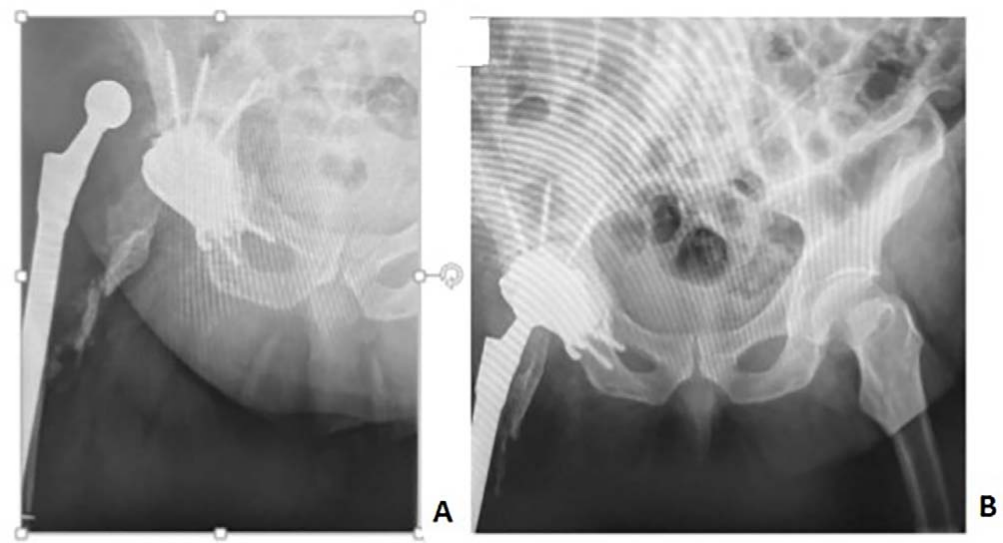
3D implant dislocation. (A) X-rays of the right hip joint with implant dislocation. (B) X-rays of pelvic bones, after open reduction of dislocation with increasing endoprosthesis head length.Early periprosthetic infection (3 cases, 6.2%); in all three cases, debridement was performed; and at the time of writing the manuscript of this article there were no signs of relapse and loosening of components in these patients.
